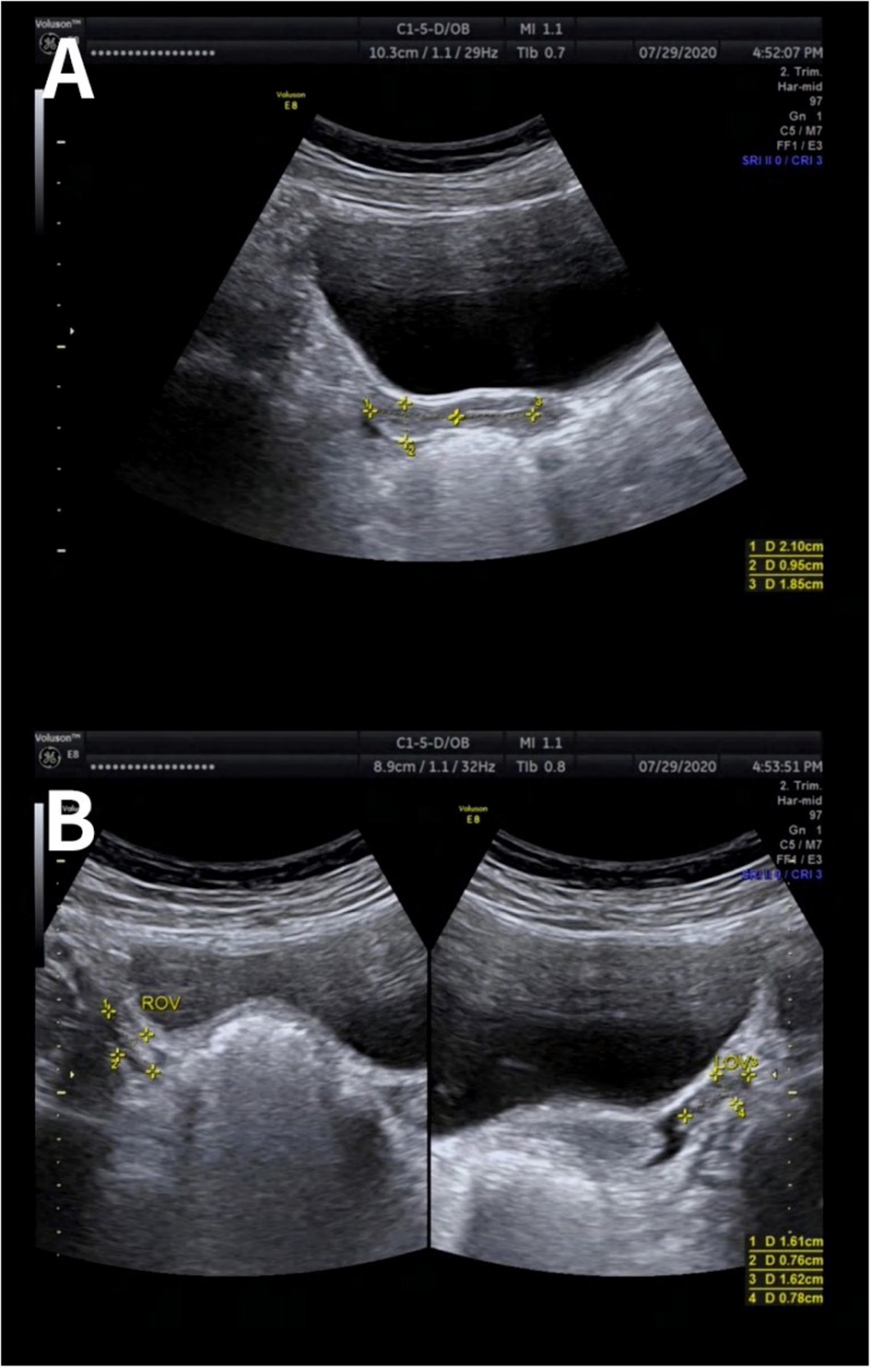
Ultrasonography image of uterus and ovaries. Transabdominal ultrasound showed small uterus (2.1 × 1.5 × 1.0 cm) with visible endometrial lining (A). Both ovaries were measured as 1.6 × 0.8 cm (B).
